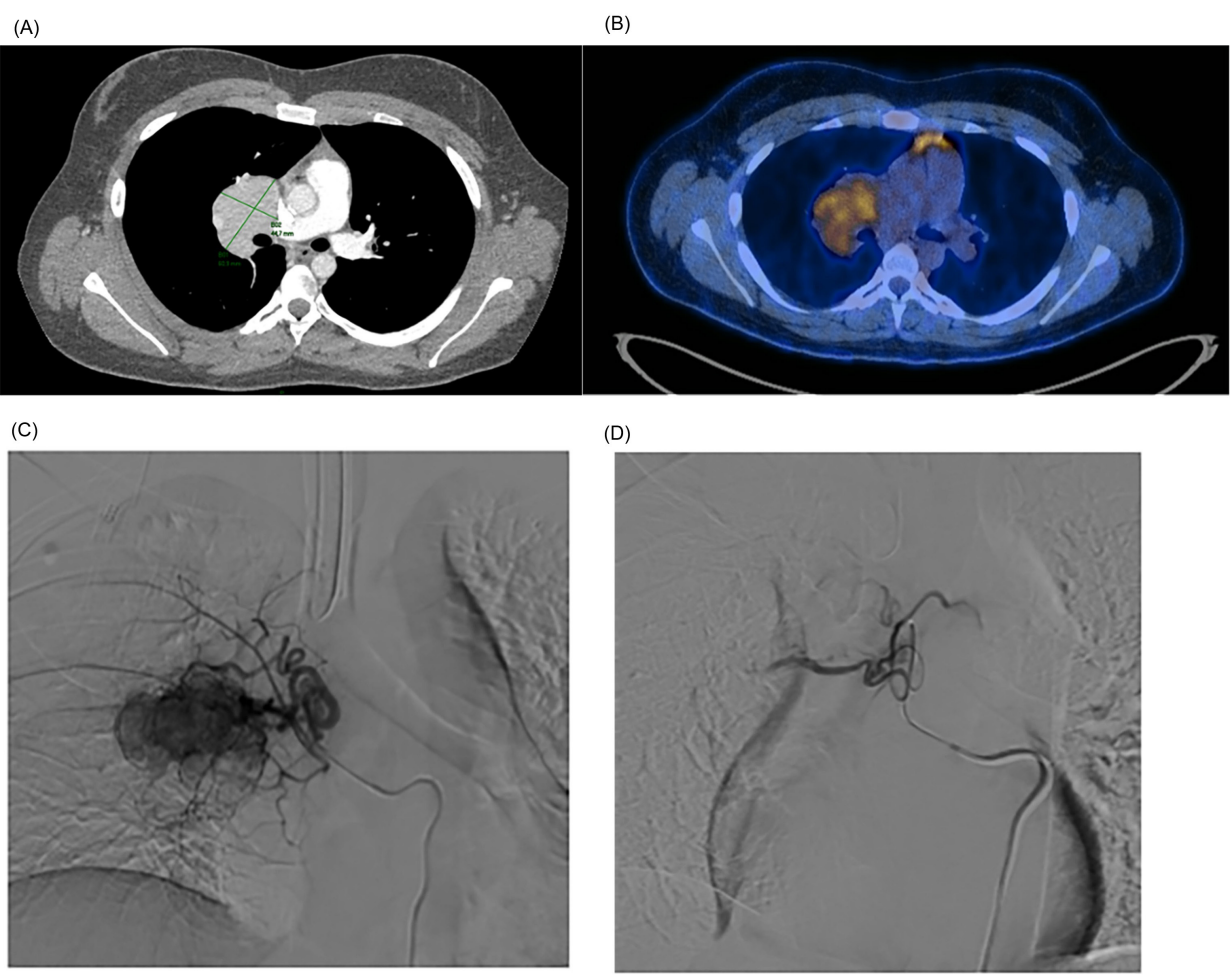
(A) Contrast-enhanced CT with axial section, at the level of the bifurcation of the pulmonary artery and the main bronchi, showing the mass in the right pulmonary hilum, measuring 6.1 × 4.5 cm; (B) PET-CT axial section with fusion of metabolic images with fluorodeoxyglucose-18F (FDG) showing radiopharmaceutical uptake with SUV of up to 4.5; (C) Selective catheterization of the right bronchial artery ostium with a 5 Fr Mikaelson catheter; (D) Angiographic control after embolization with Embosphere® Merit 300-500 microns microspheres, showing an outcome with contrast stasis in the feeding trunk branch.
